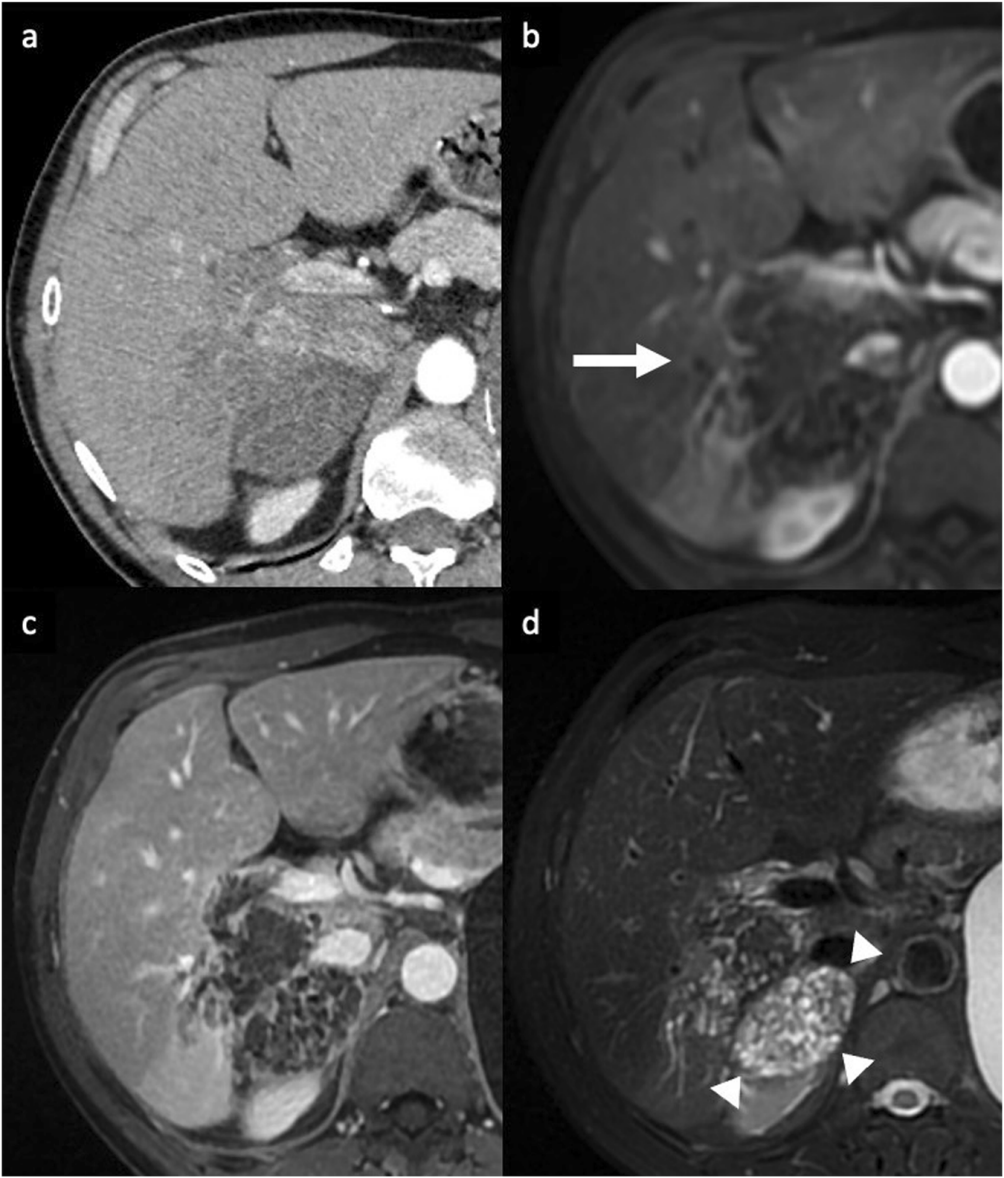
Alveolar echinococcosis in a 61-year-old man who presented with right upper quadrant pain and hypereosinophilia. a Contrast-enhanced CT shows a subcapsular exophytic liver lesion in the right hepatic lobe, containing hypoattenuating areas of necrosis and thin-rim APHE. On post-contrast MRI using an extracellular contrast agent, the lesion shows (b) a rim APHE (arrow) that persisted on (c) portal venous phases. d On axial T2-weighted MRI, the lesion appears slightly hyperintense, with the coalescence of multiple small cystic lesions (arrowheads) in a single larger cavity (“cluster sign”)
